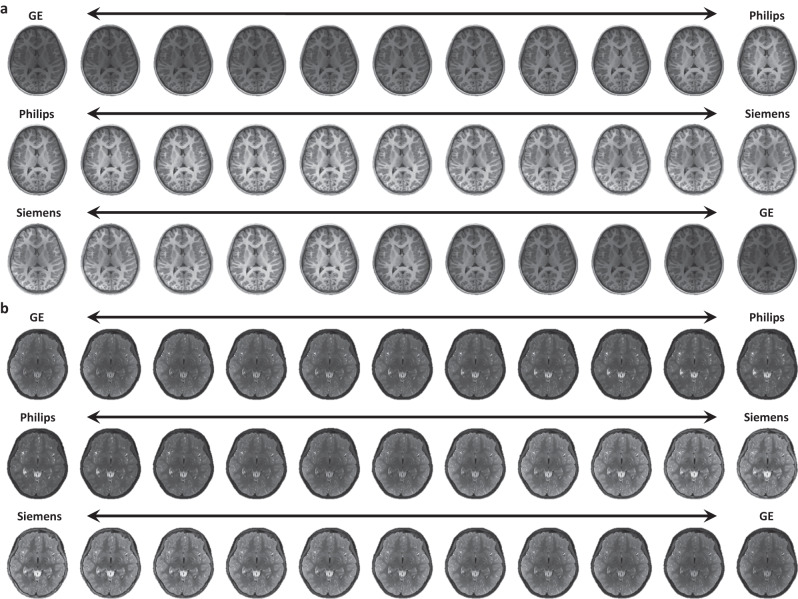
Continuous harmonization. Continuous harmonization of a T1-weighted images, and b T2-weighted images between two sites, demonstrating that MURD smoothly changes image contrasts without altering anatomical details thanks to its ability to disentangle content and style information.
